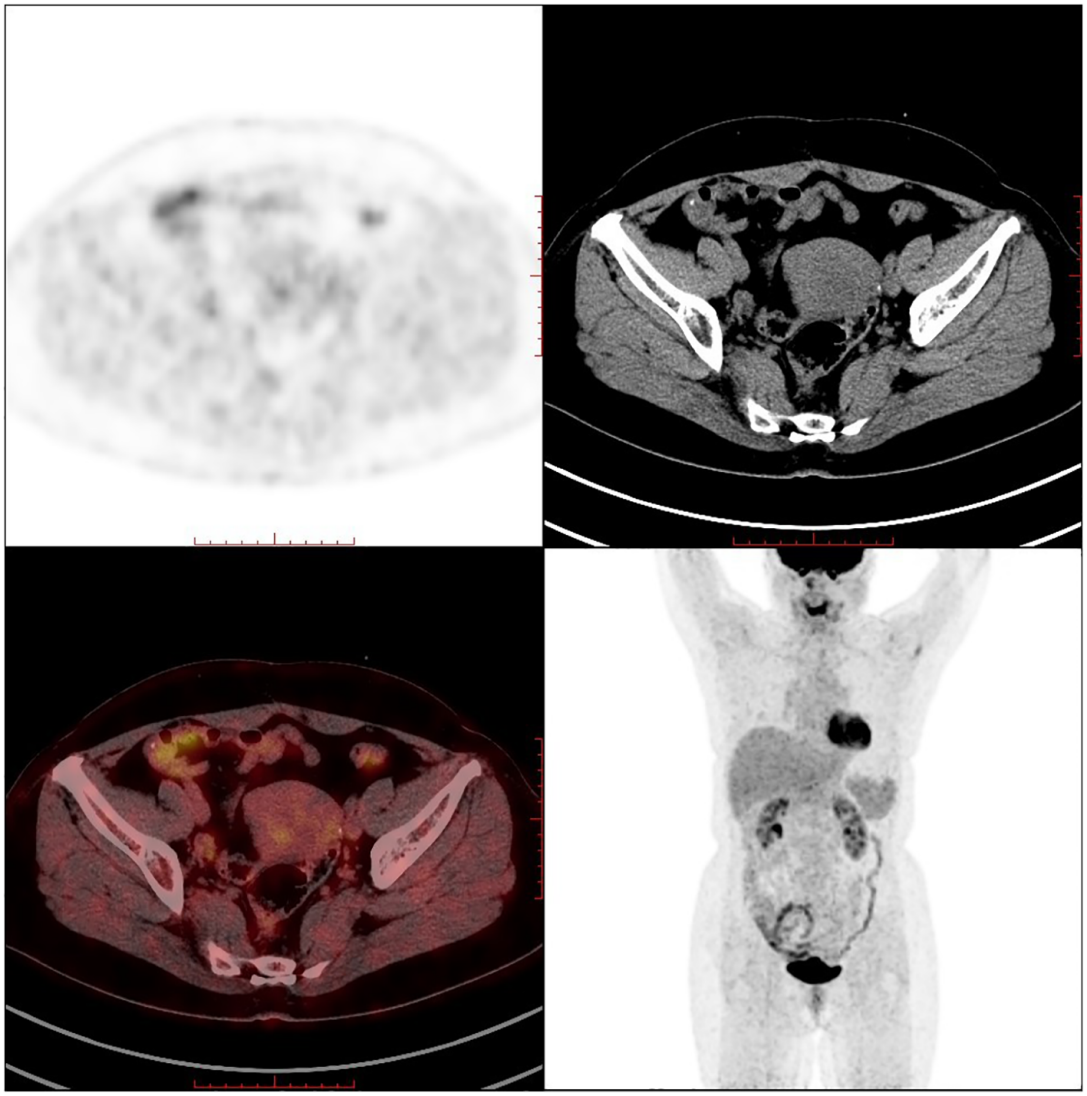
PET-CT after ovarian lymphoma surgery (Case 3).
